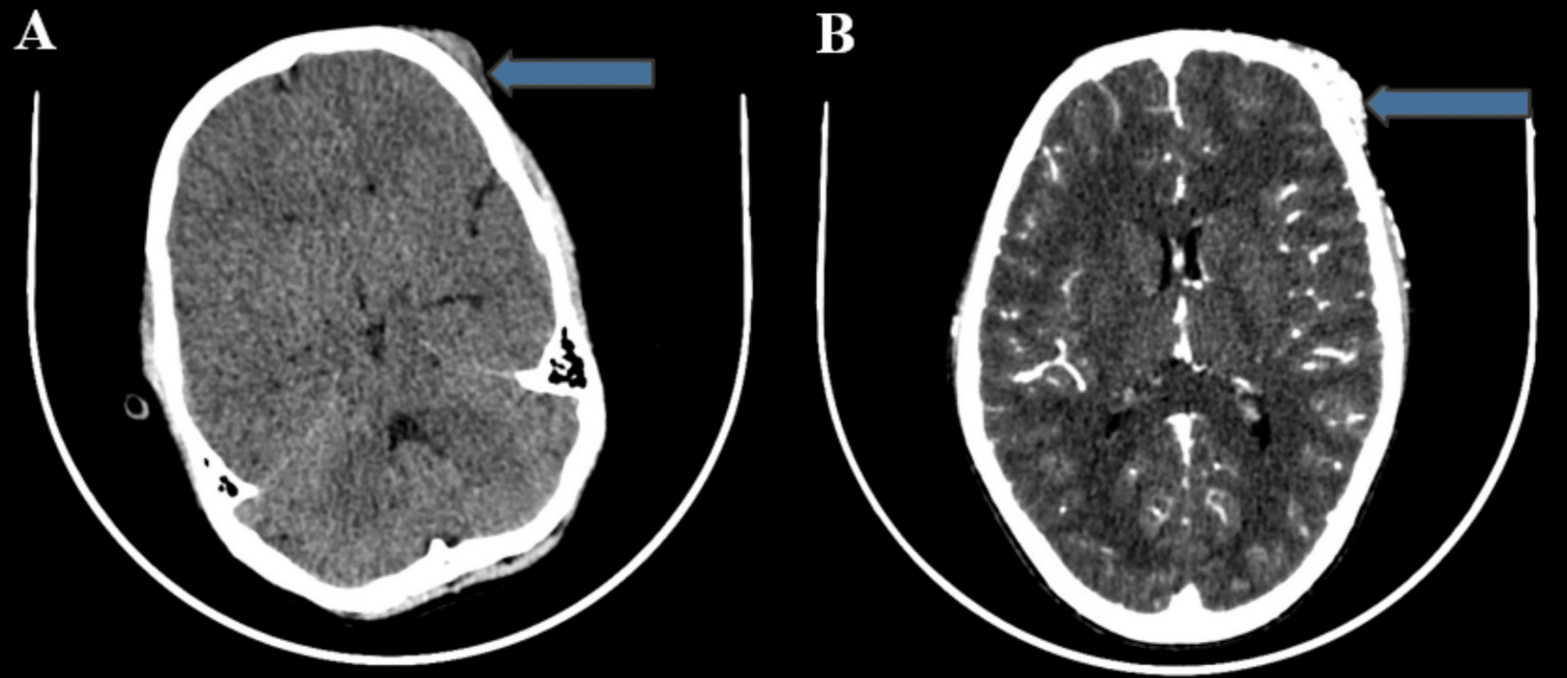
(A) CT brain (plain) and (B) CT carotid angiography showing a soft tissue density lesion in the subcutaneous plane of the scalp in the left frontal region with significant enhancement (blue arrow). This finding is suggestive of an arteriovenous malformation.
